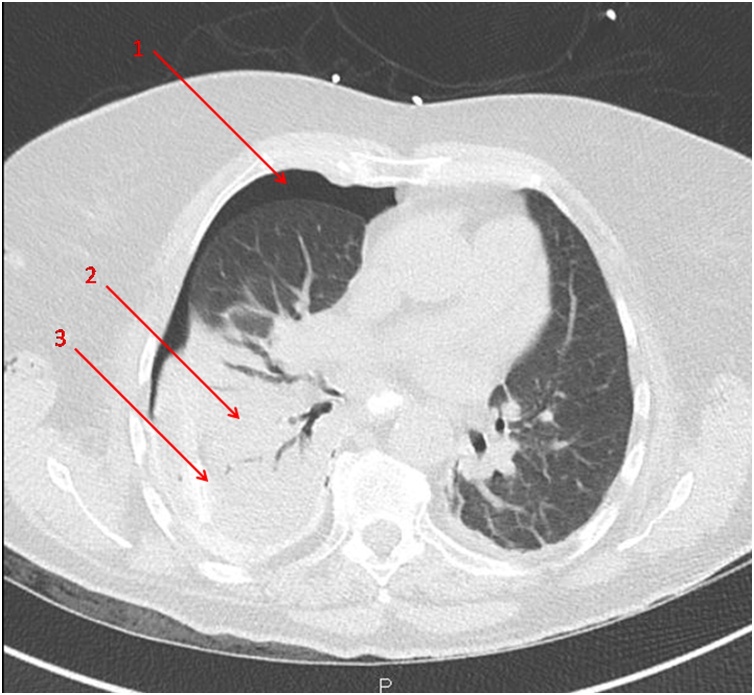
Preoperative CT scan. 1 : pneumothorax 2 : atelectasia 3 : pleural effusion.
